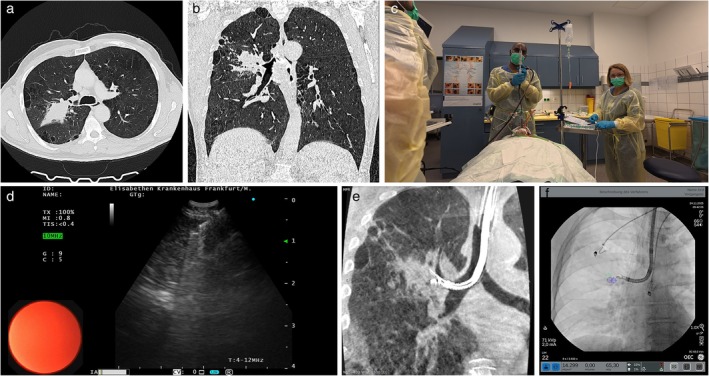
(a) Axial CT‐Scan showing a lesion in the right upper lobe. (b) Coronal CT‐Scan showing a lesion in the right upper lobe. (c) EBUS‐TBNA being carried out with 25G needle. (d) EBUS image of cryoprobe in the Lymph node. (e) CBCT‐Image of EBUS‐Bronchoscope in the right upper lobe. (f) Augmented fluoroscopy after CBCT showing TBNA needle in the upper lobe lesion.
